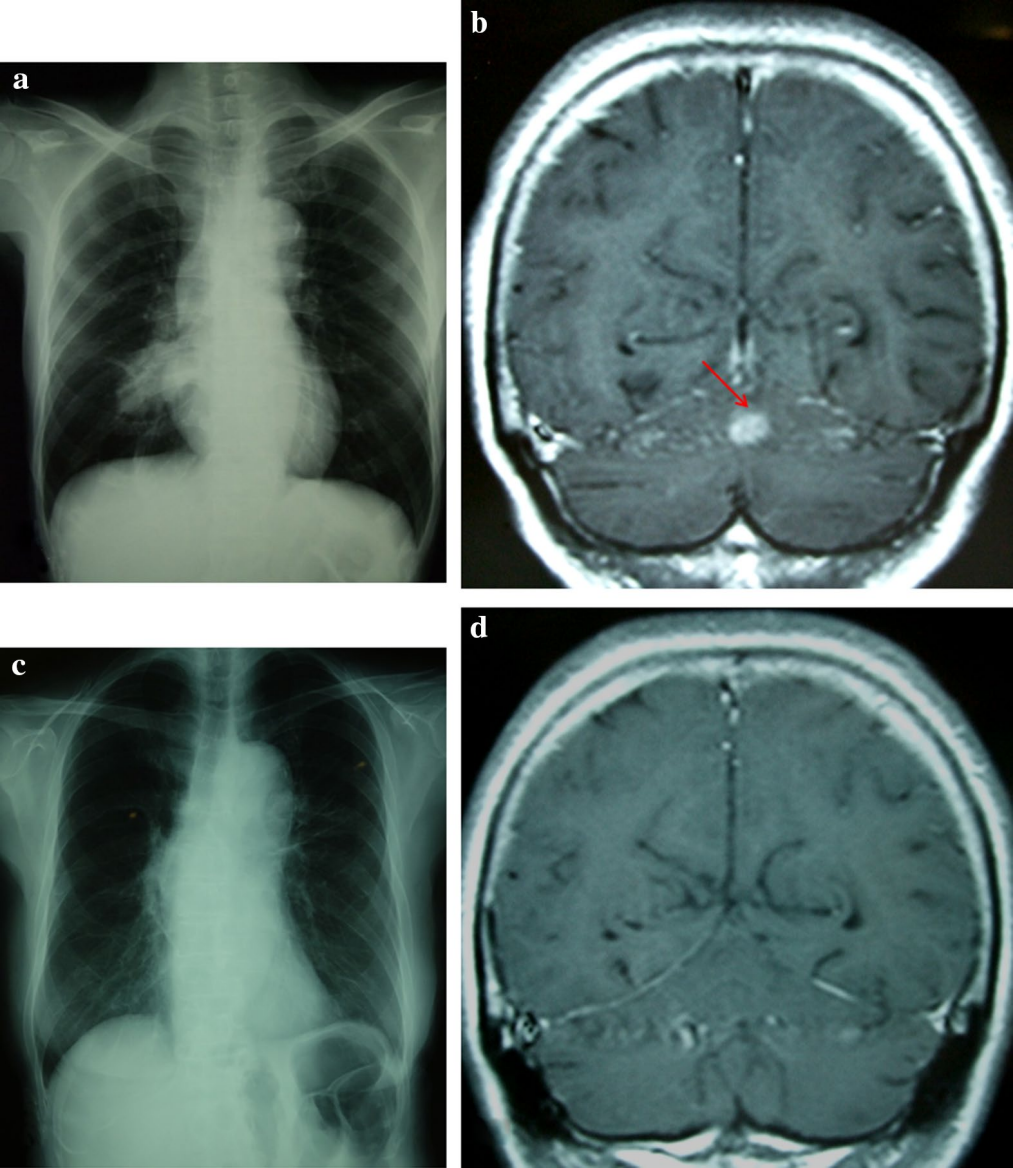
Chest X-ray examination and coronal T1-weighted magnetic resonance imaging with gadolinium. a
